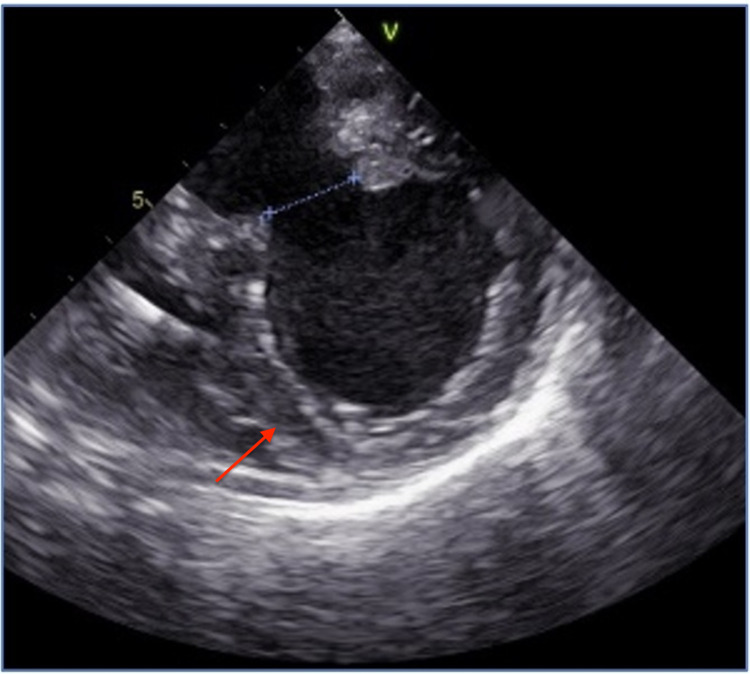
Transesophageal echocardiogram showing a large left ventricular aneurysm.
